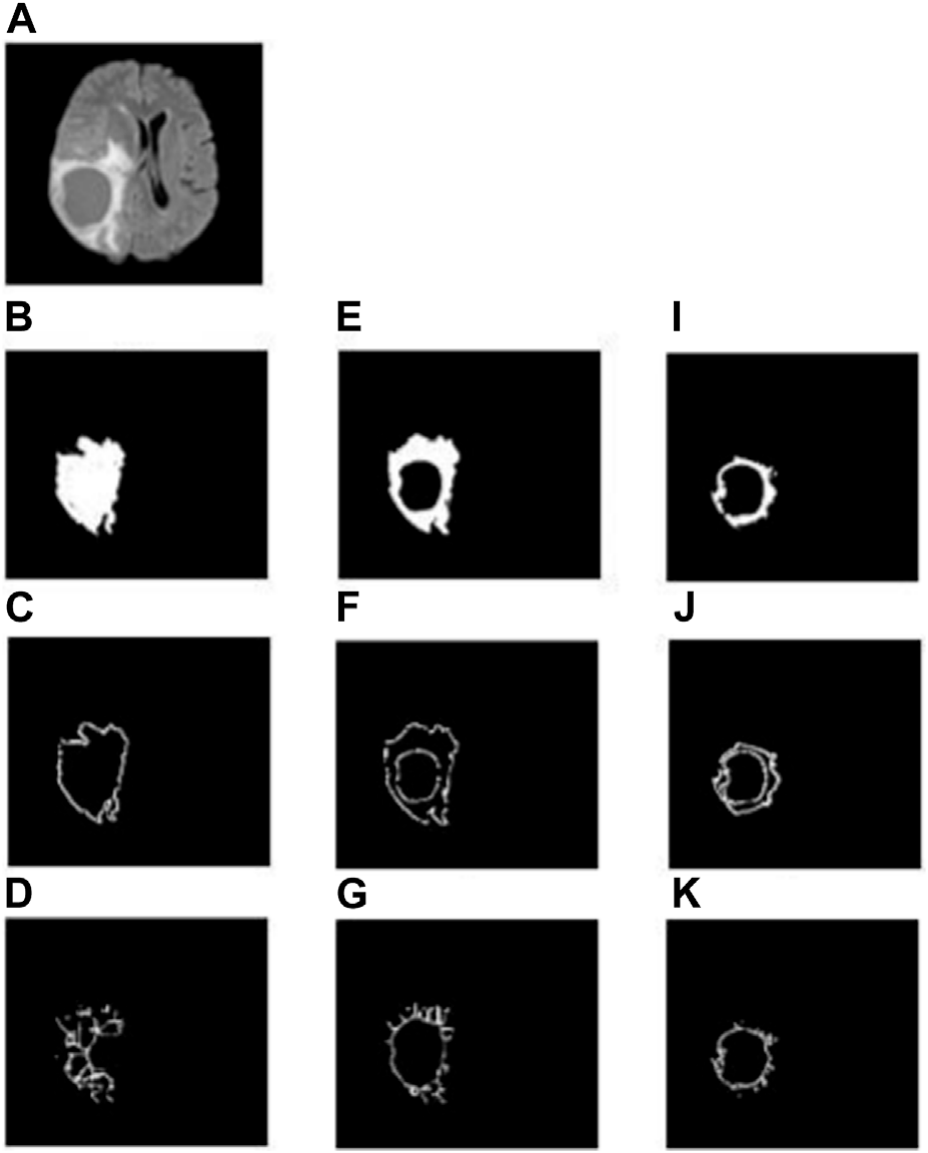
(A) FLAIR image of a typical HGG patient, (B–D) general structure, boundary, and skeleton of the whole tumor region, (E–G) general structure, boundary, and skeleton of the edema region, (H–J) general structure, boundary, and skeleton of the enhanced tumor region, respectively.
